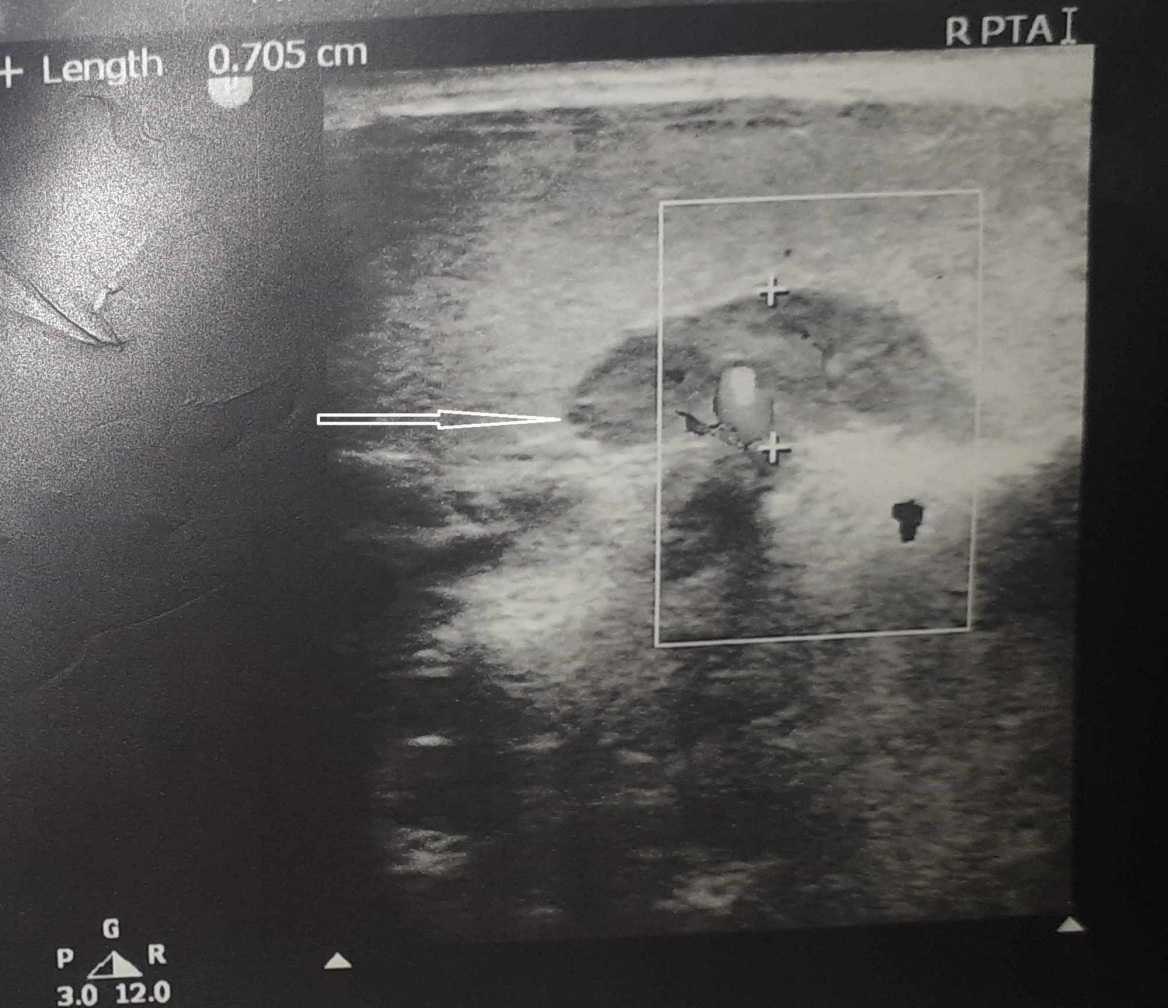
High-resolution ultrasound image showing a well-defined hypoechoic mass of 14x7 mm in diameter (white arrow).
